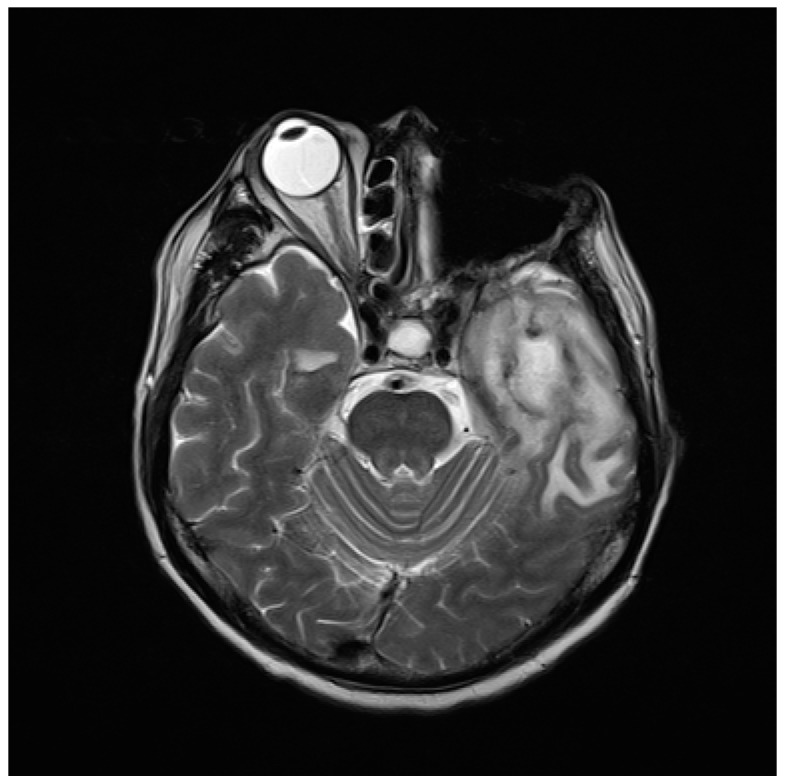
Magnetic resonance image (MRI) of brain showing heterogeneous enhancement with focal rim enhancing cystic lesions in left anterior temporal lobe.
